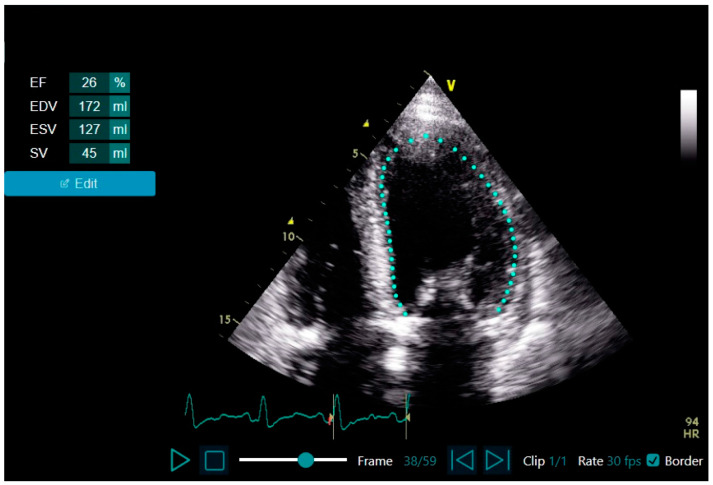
The LVivo EF: AI-based tool for automated LVEF assessment from apical 4-chamber view echocardiographic clips. Abbreviations. AI, artificial intelligence; LVEF, left ventricular ejection fraction.
